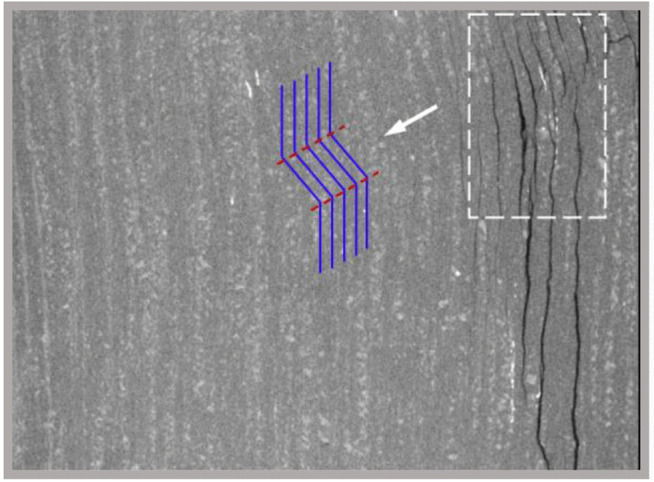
Localized kinking revealed by CT scanning.
